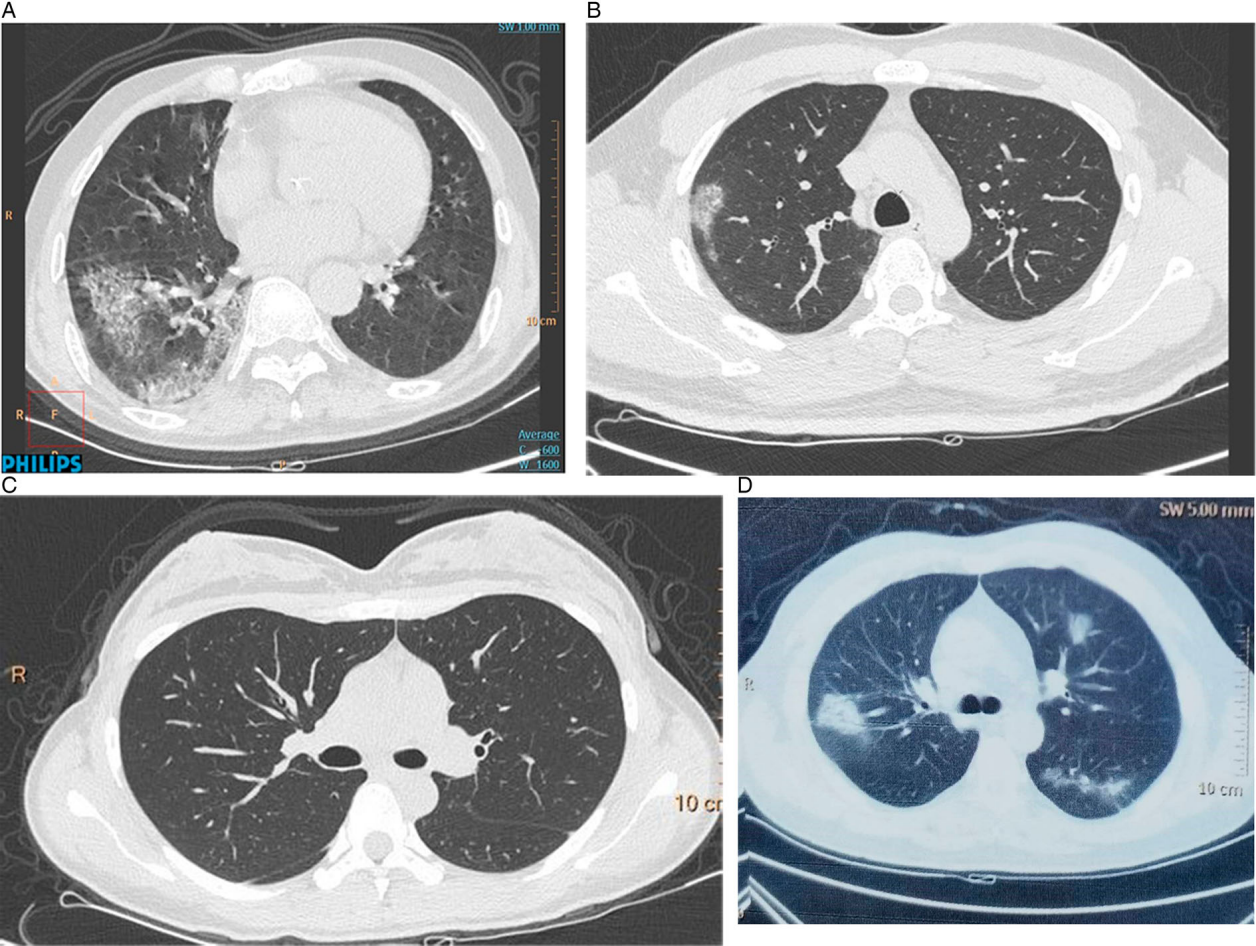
Lung computed tomography (CT) images of case 7 and case 8’s families. (A) The CT of their grandpa, who was 72-year-old and admitted for symptoms of high fever for 3 days. (B) CT scan of case their father, a 38-year-old patient admitted for symptoms of high fever, shortness of breath and fatigue for 10 days on his admission day. (C) Lung CT image of their mother, a 32-year-old female patient admitted for positive result of PCR (C). (D) Chest CT of the twins’ grandma, 65 years old and had fever, dry cough and chest pain for 6 days before admission.
